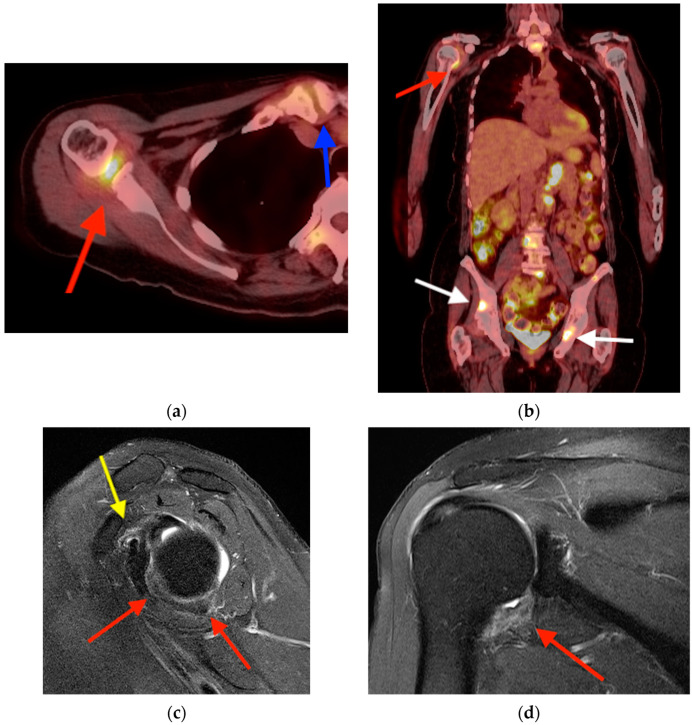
Axial (a) and coronal (b) FDG PET/CT images obtained in a 58-year-old woman with metastatic renal cell carcinoma show focal uptake in the right axillary recess (red arrows), along with additional areas of focal uptake at the site of known metastatic lesions (white arrows). There is osteoarthritis-related focal uptake at the right sternoclavicular joint (blue arrow). Axial (c) and coronal (d) T2-weighted MRI images show thickening of the joint capsule and inferior glenohumeral ligament at the axillary recess (red arrows) and effacement/obliteration of the coracoid fat and rotator interval with associated thickening of the glenohumeral ligament (yellow arrow), characteristic of adhesive capsulitis. FDG PET/CT = fluorine-18 fluorodeoxyglucose positron emission tomography/computed tomography. MRI = magnetic resonance imaging.
